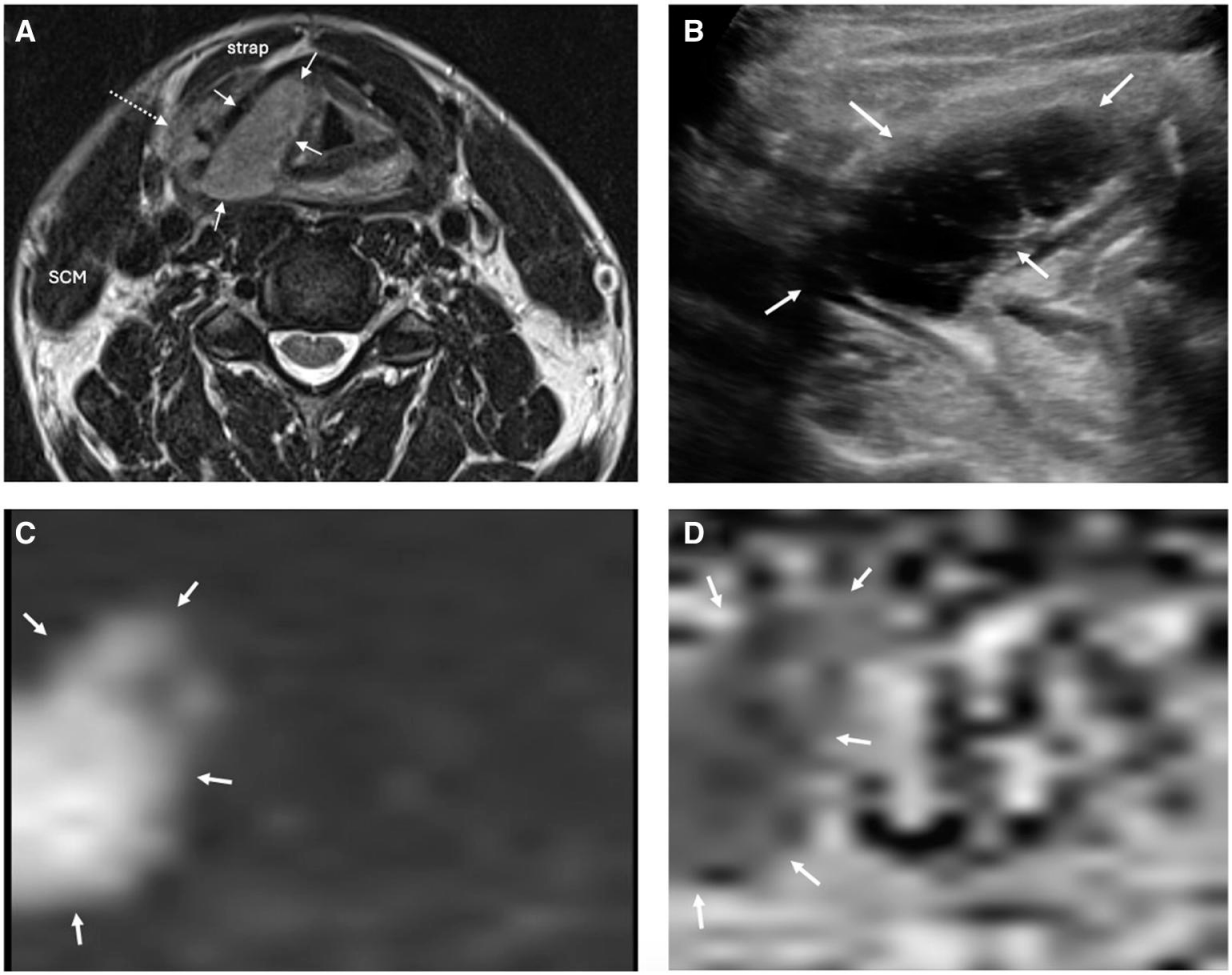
Right thyroid lamina EMP in MM relapse (solid thin white arrows). (A) Axial T2-weighted MRI shows an expansile, intermediate signal mass centred on and eroding the low-signal ossified right thyroid cartilage. There is a superficial component elevating the right strap muscles (dotted arrows) and an endolaryngeal component partially effacing the right paraglottic fat. (B) Transverse high-resolution US image (curvilinear C3-10 MHz probe) shows the hypoechoic mass centred on the thyroid cartilage. Real-time US indicated that the cord was still mobile and facilitated percutaneous image-guided tissue sampling of the superficial component. (C and D) DWI and ADC sequences demonstrating restricted diffusion.
